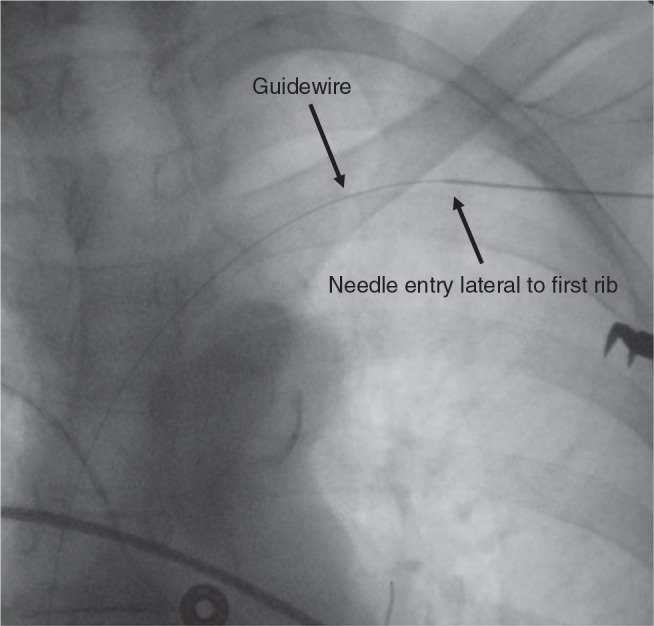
Fluoroscopic view of the point at which the axillary vein is entered using ultrasound (US) guidance. The point at which the tip of a 21-gauge needle was advanced through the anterior wall of the axillary vein with direct US is marked with an arrow. The location of the 0.018-in wire, which was passed through the needle and advanced to the level of the inferior vena cava, is also marked with an arrow.
